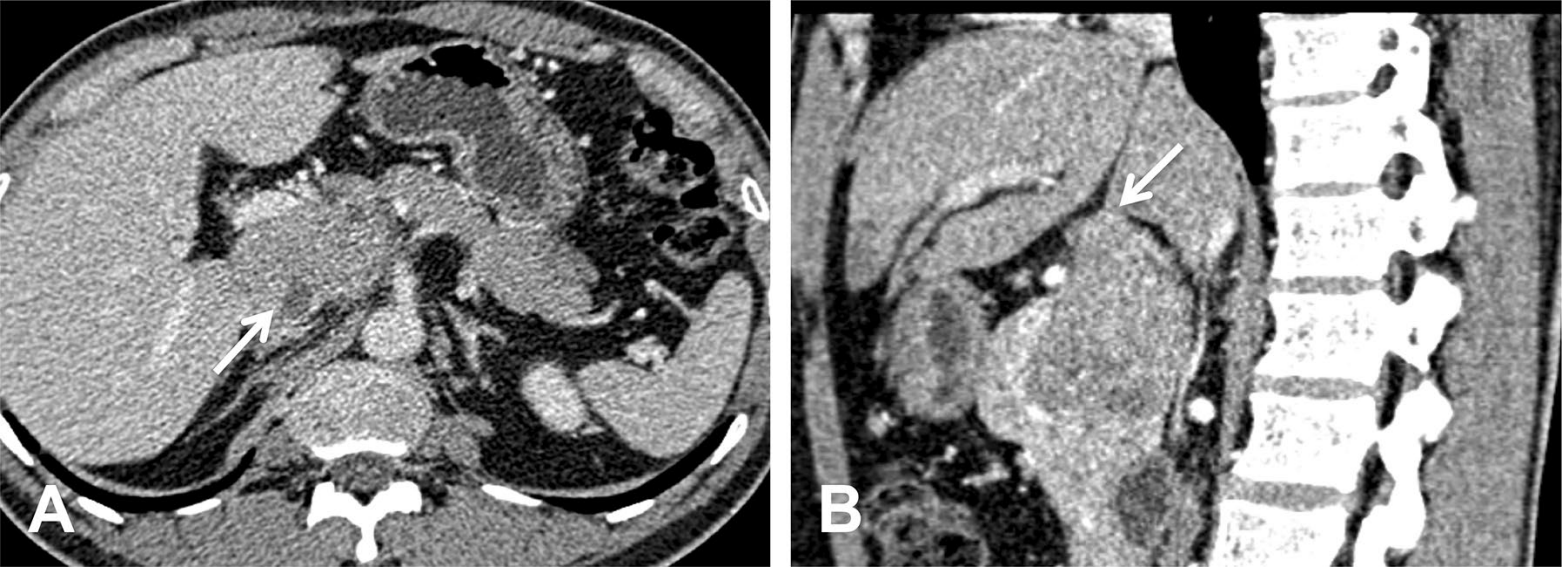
CT image of a 37-year-old man with pathologically confirmed PB. (A) In the VP view, the mass appears as an isodense mass with well-defined cystic degeneration in the peripheral portion of the mass. (B) The sagittal MPR images show that the tumor exhibits an exophytic growth pattern, similar to branches growing outward from a tree trunk, with a pointed head deep into the hilum of the liver (arrow).
